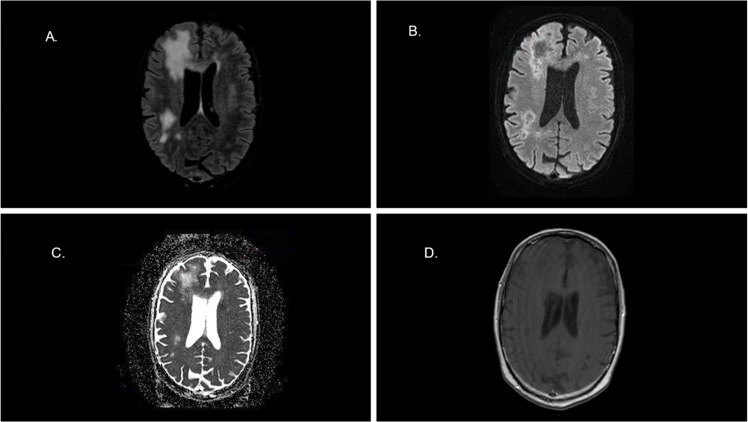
(A) 3D-FLAIR image shows areas of hyperintense signal in the white matter of the right hemisphere involving the U fibers. (B) DWI and respective ADC map (C) show that lesions manifest a hypersignal wavefront spreading without diffusion restriction (lack of hyposignal in ADC map). This finding indicates an activee myelinoclastic process at the wavefront. (D) The axial T1 image after contrast medium administration shows no enhancement of the lesions.
